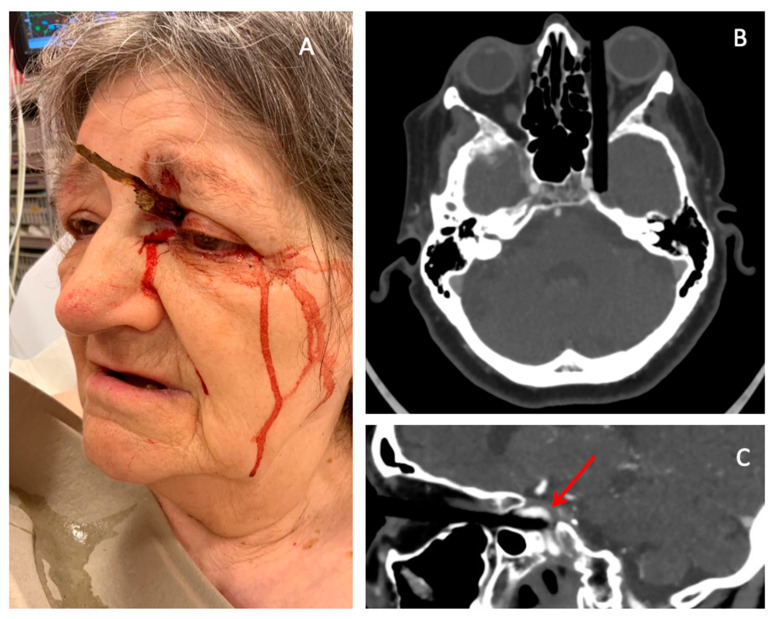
Example of a penetrating orbital injury caused by a tree branch. (A) Picture of the patient at presentation in the emergency room. (B) Transversal computed tomography view of the patient’s skull. (C) Sagittal view showing the tree branch located between the intracavernous segment of the internal carotid artery and the temporal lobe (Arrow). Credits Flament, J.; Moraine, A [8].
